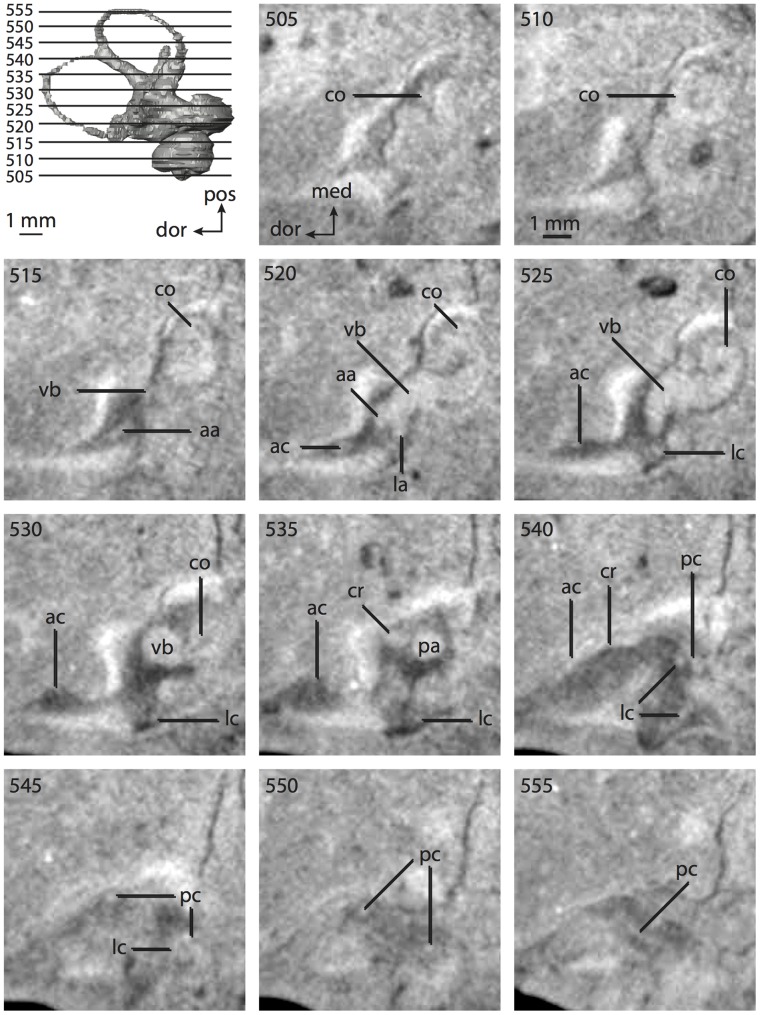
CT slices through ear region of*Bathygenys reevesi*. Abbreviations listed at the end of the Materials and Methods section.
